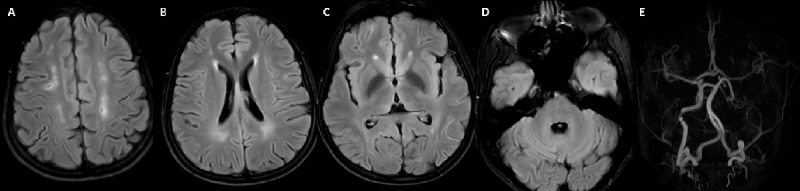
MRI of Patient-5. FLAIR axial sections (A-D) show chronic multiple watershed infracts in bilateral internal carotid artery territory (A) and subtle periventricular white matter signal changes (B). Deep grey nuclei (C), dentate and cerebellar white matter (D) appear normal. Time-of-flight MR angiography (E) show complete occlusion of the intracranial segment of the left internal carotid artery.
